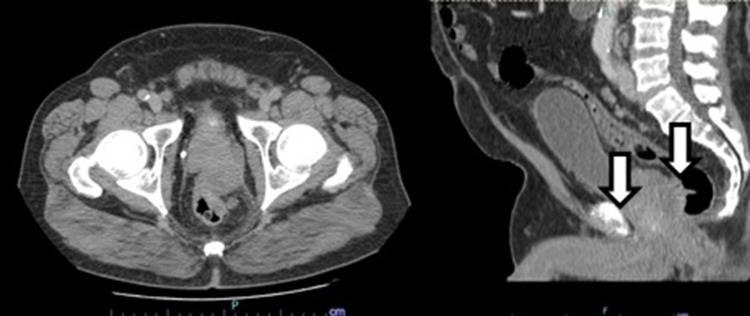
Computed tomography axial plane at pelvic level with large heterogenic prostatic mass (59 x 35 x 33 mm) with loss of cleavage planes with the anterior and left rectum walls.
